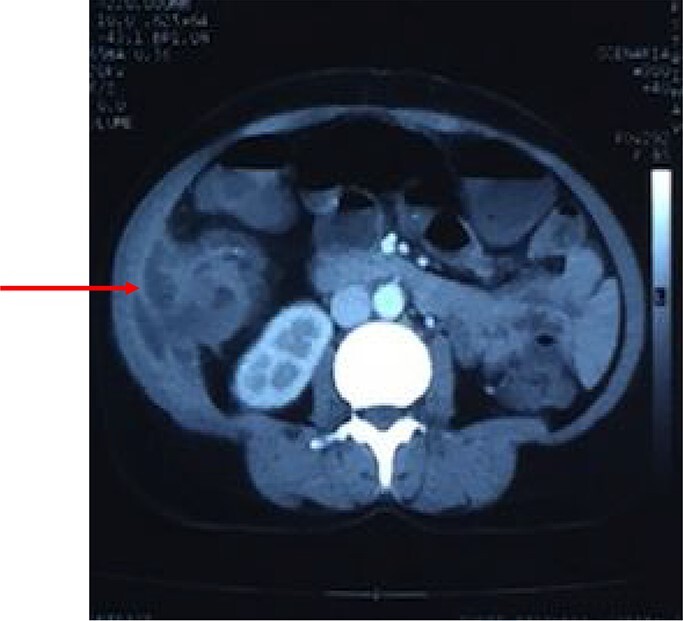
Computed tomography of the abdomen showing perforation of the tumor through muscles of lateral abdominal wall with abscess formation as seen on CT scan.
